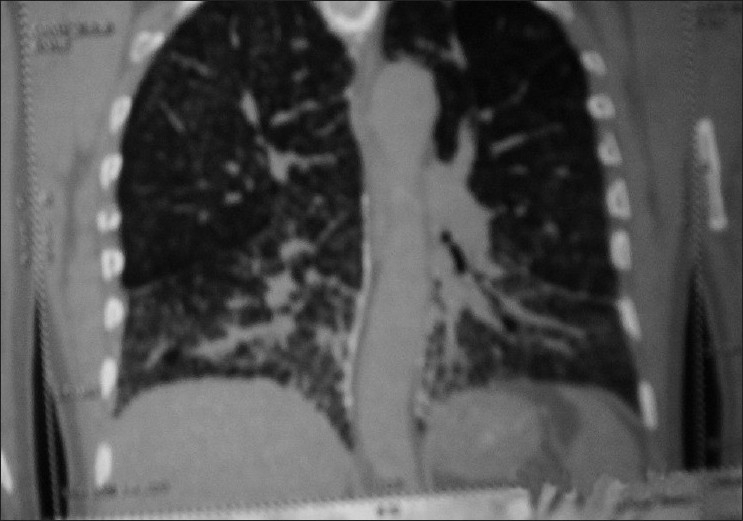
CT scan revealing diffuse bilateral calcified fine nodular pattern with extensive septal thickening
